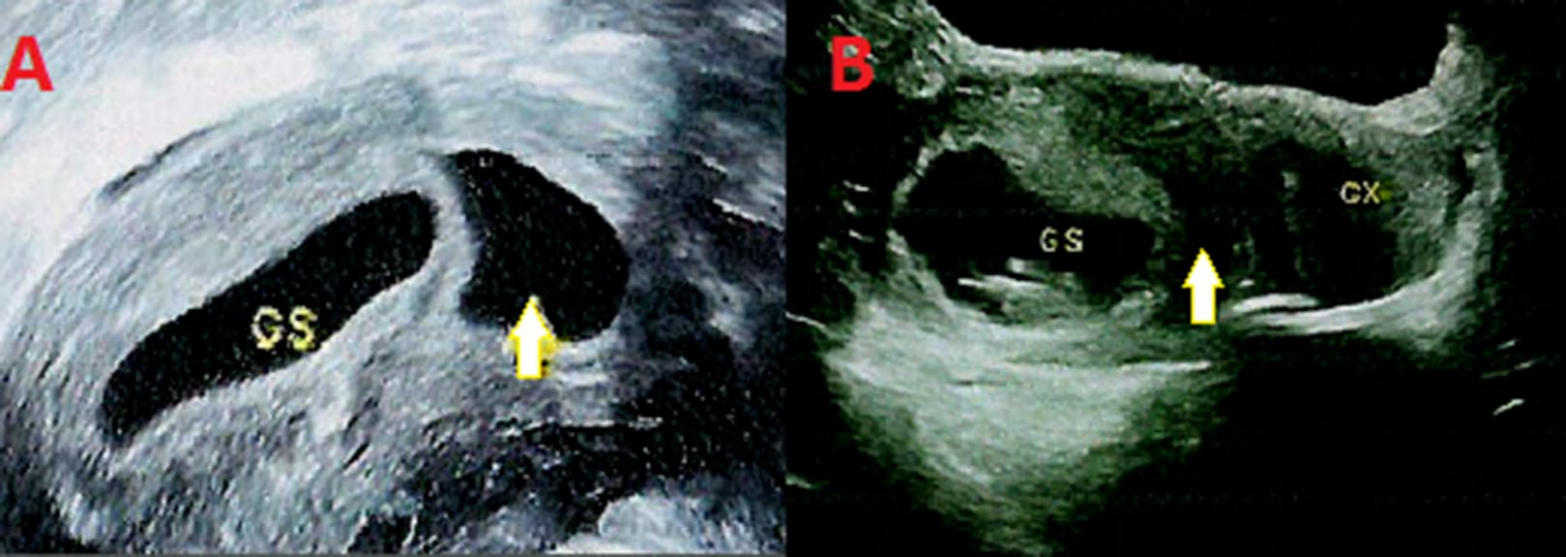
The ultrasound results during early pregnancy. (A) Ultrasound image at 7^+2^ weeks gestation. (B) Ultrasound image at 9^+2^ weeks gestation. GS: Gestation sac. The area indicated by the yellow arrow represents the echoless zone
